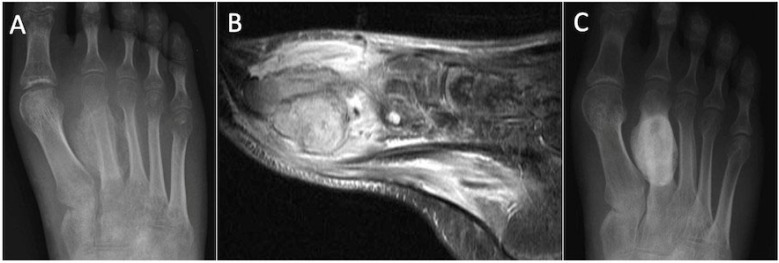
Dorsoplantar radiograph (A) and STIR sagittal MRI (B) demonstrating florid reactive periostitis and extensive local osseous and soft tissue oedema. Subsequent radiograph (C) demonstrates consolidation of the periostitis ossificans.
